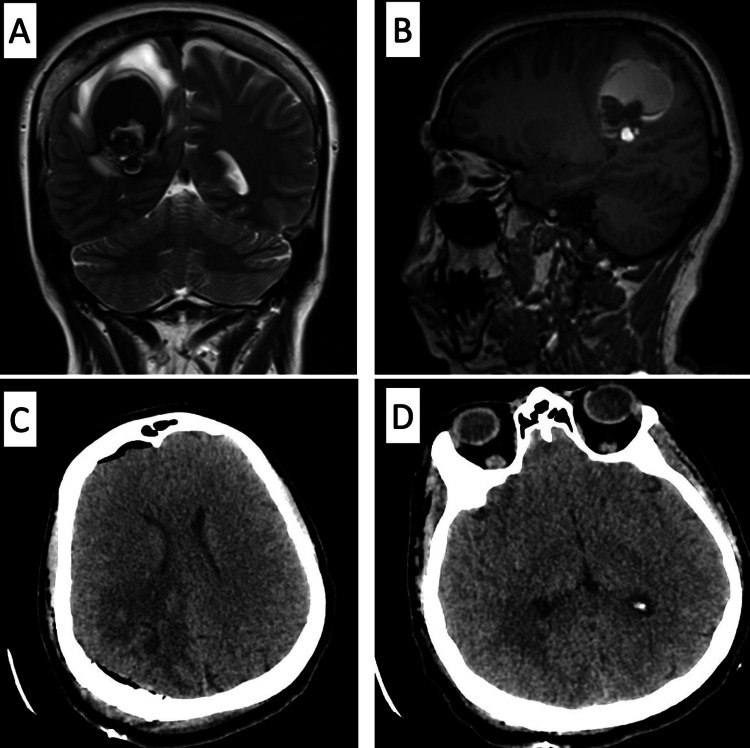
CT and MRI scans The lesion showed a fluid-fluid level, which is best displayed by the coronal T2-weighted image (A) and sagittal T1-weighted image (B). Status post excision of the lesion with immediate post-operative changes are noted on an unenhanced CT scan of the brain (C and D).
